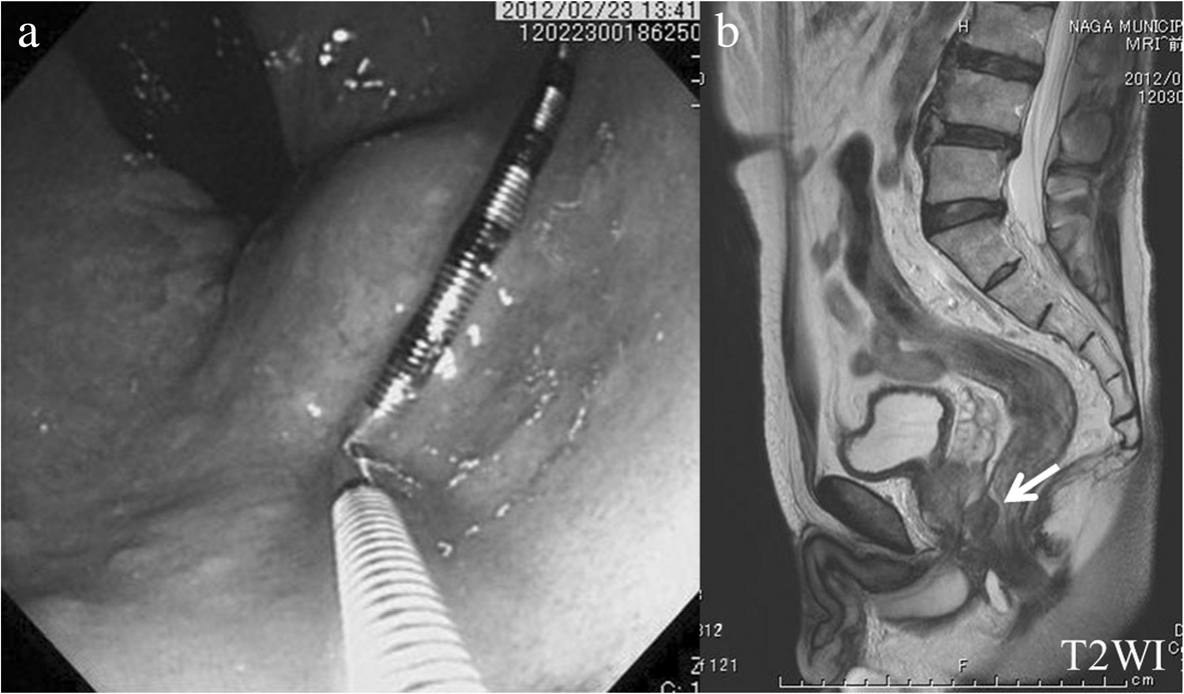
Colonoscopy and a T2 sagittal magnetic resonance image showed a mass on the anterior wall of the rectum. (a) Colonoscopy disclosed a bulge which probably originated in the submucosal layer. (b) A T2 sagittal magnetic resonance image demonstrated a mass on the anterior wall of the rectum without any invasion to the prostate (arrow).
